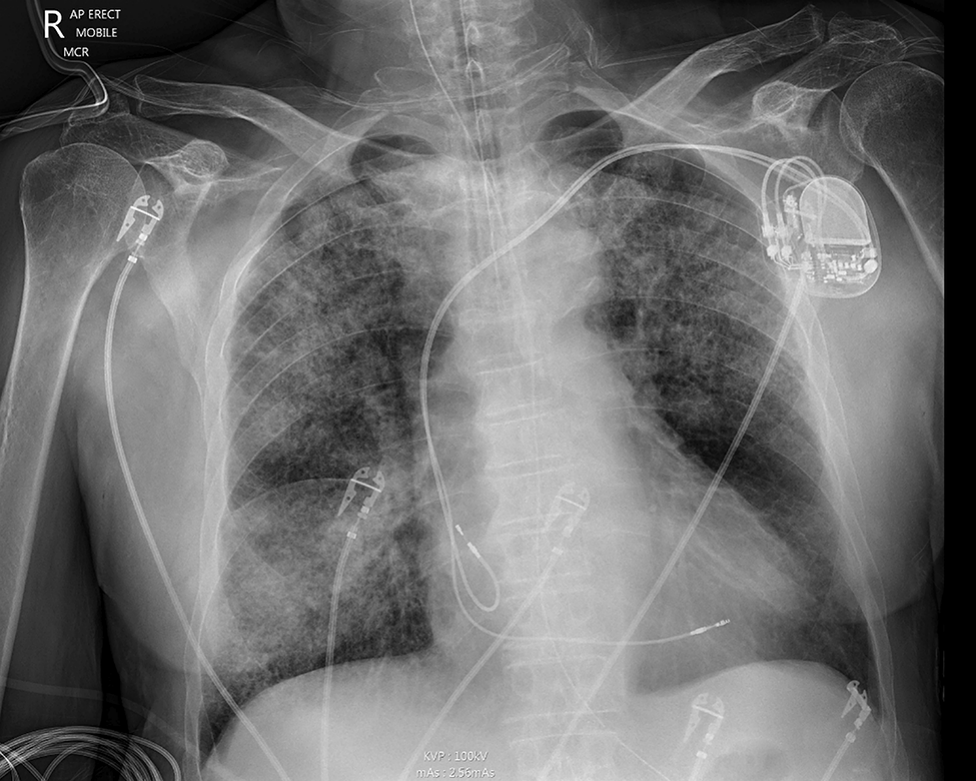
Chest radiograph of a patient (patient 7) showing right upper lobe opacification in study of acute Q fever infections in the intensive care unit in tropical Australia, 2015–2023. The patient required mechanical ventilation for 72 hours.
